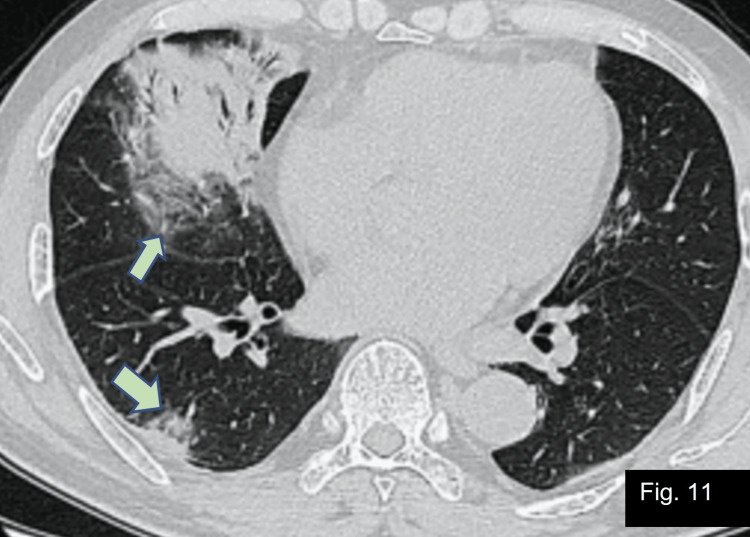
CT chest after 2-3 weeks of symptom onset Dissipation stage (2-3 weeks after symptom onset). There are very few lesions. Image source: Reference no. [25]
